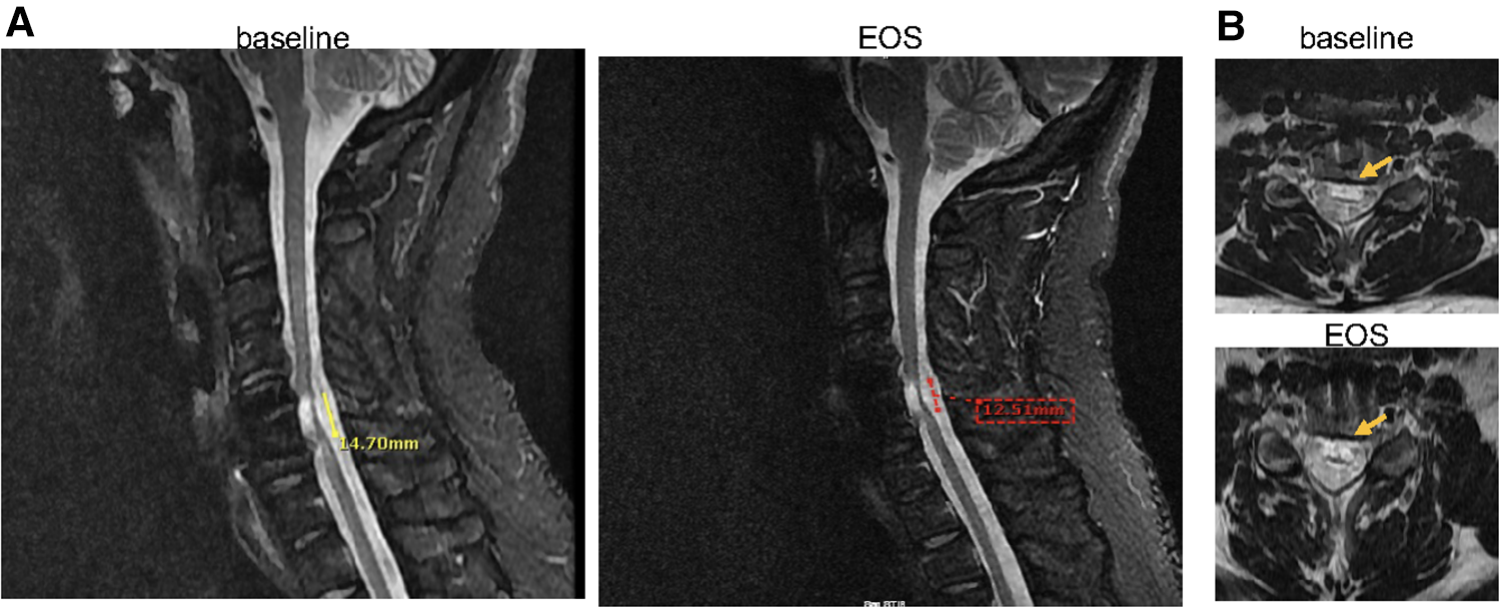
(A) Sagittal MRI images (baseline vs. EOS) shows mildly decreased craniocaudal length of the area of T2 abnormality (14.70 mm vs. 12.51 mm), and (B) axial view (baseline vs. EOS) shows minimal increase in the non-edematous portion of the cord at the EOS (post 34 infusions, at month 30) compared to baseline (represented by arrows). EOS, end of study.
